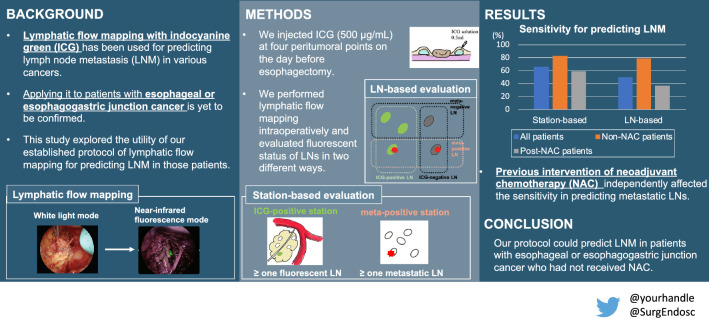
Esophageal cancer has a poor prognosis, even though various treatment strategies have been established [1]. The presence of lymph node metastasis (LNM) is a critical prognostic factor for esophageal cancer [2], making the accurate prediction of metastasis crucial for planning an appropriate treatment course. Preoperatively, LNM is most commonly predicted using the size of lymph nodes (LNs), determined using computed tomography. However, computed tomography has a poor ability to detect LNMs. The most recent edition of the Japanese Classification of Esophageal Cancer states that—using cutoff values of 6 to 8 mm for the nodal diameter—the sensitivity of computed tomography for detecting LNM in patients with cT2-4 esophageal cancer ranges from 27.8 to 65.2% for mediastinal LNs and 52.3% to 81.8% for abdominal LNs [3]. Almost one-third of metastatic LNs reportedly have diameters of less than 5 mm [4]; thus, the features of LNs visualized using computed tomography may provide limited information for accurately predicting LNM in esophageal cancer.
